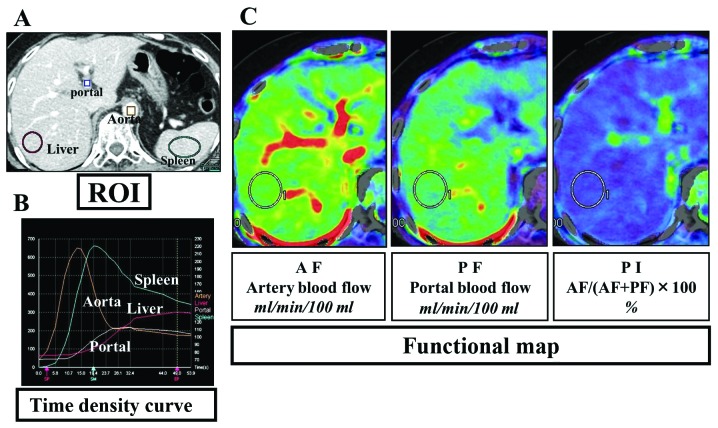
Imaging analysis of hepatic CT perfusion. In order to obtain perfusion parameters, it was necessary to set the region of interest (ROI) in (A) four sites. (B) The time density curve and (C) parameters were calculated and displayed as a functional color map using a software program. A round or oval-shaped ROI was placed on the right hepatic lobe on the functional map, so that the ROI would be as large as possible while avoiding vessels and artifacts.
